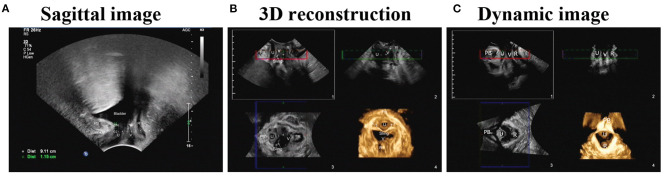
Real-time dynamic ultrasound images of the mobility of bladder neck, urethra and sling at rest or valsalva maneuver. (A) Sagittal transperineal ultrasound image demonstrating the anatomic structure of the pelvic. PB, Pubic bone, BN, bladder neck; U, urethra; V, vagina; R, rectum. (B) Axial 3D reconstruction and dynamic ultrasound technologies allows the assessment of sling and pelvic structures in multi imaging planes in a patient with MUS implantation through a transobturator approach. (C) Dynamic ultrasound images detected during valsalva maneuver or at rest in a female patient.
